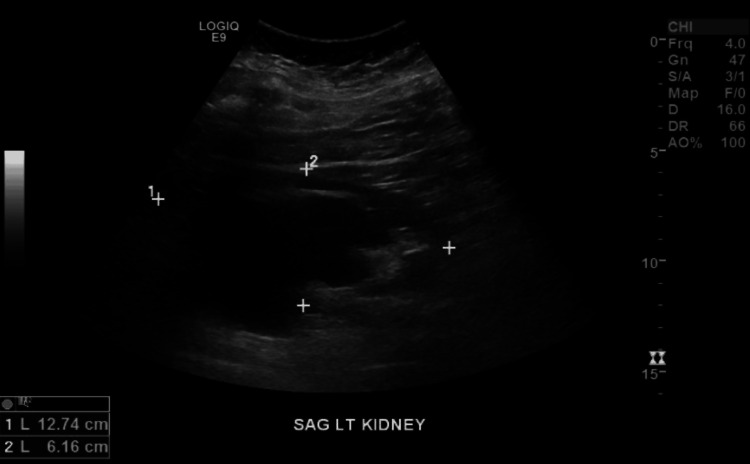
Complete renal ultrasound showed bilateral hydronephrosis, prominently worse on the left.
